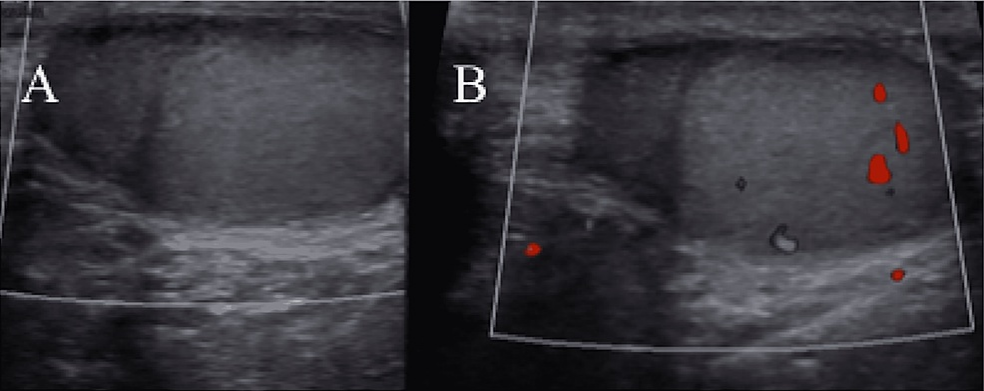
Follow-up color Doppler ultrasound that shows homogeneous left testis with a heterogeneous area with diminished blood flow related to remote insult
